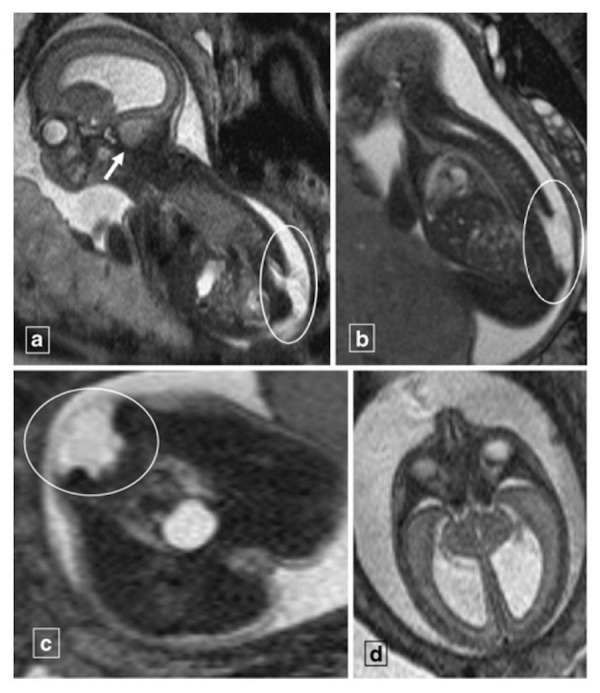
Neural tube defect with tonsillar herniation Panel A is a T2-weighted sagittal image of a 23-week gestational age fetus demonstrating a lumbosacral neural tube defect (encircled) with cerebellar tonsillar herniation (arrow). Panel B demonstrates is a T2-weighted sagittal image demonstrating a myelomeningocele (encircled) from L2 to the end of the sacrum. Panel C demonstrates the myelomeningocele (encircled) in the axial plane. Panel D is a T2-weighted axial image demonstrating hydrocephalus. The findings are consistent with Chiari II malformation. Image reprinted without changes from Nemec, et al. under the creative commons license.
